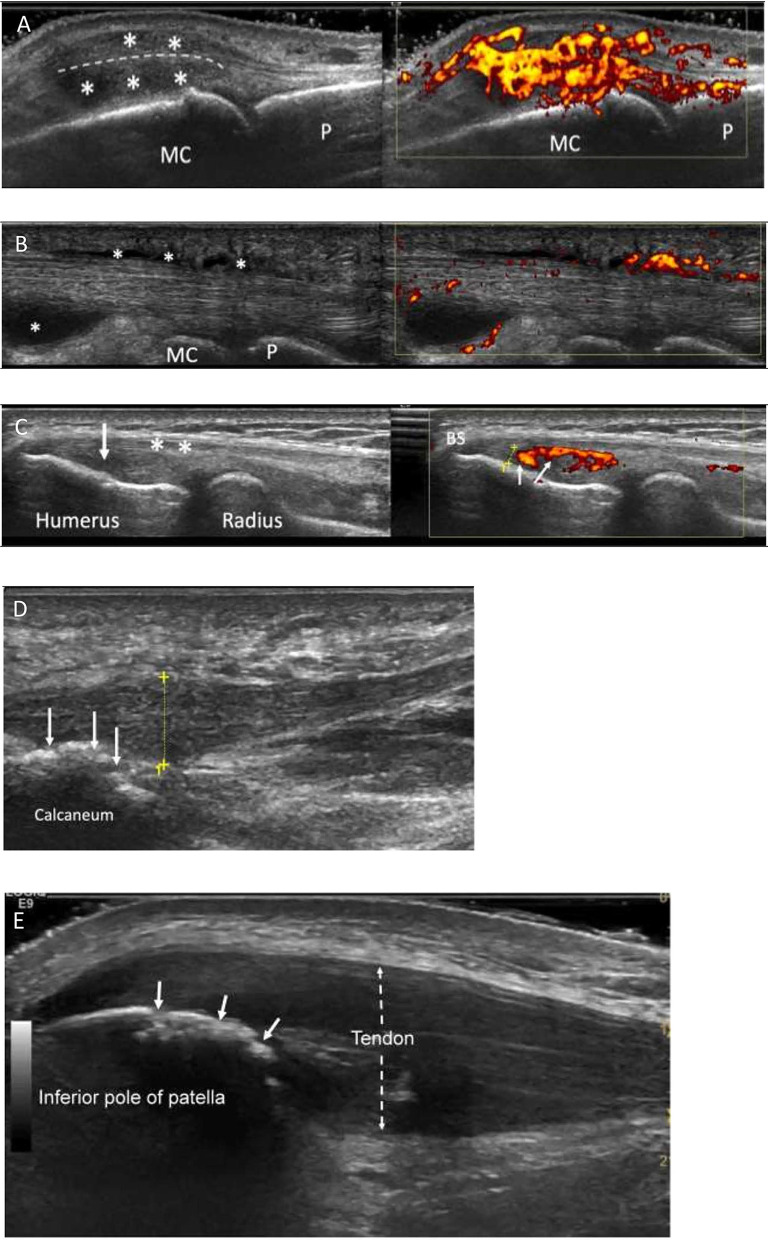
Characteristic ultrasound appearances in Psoriatic arthritis. (A) Longitudinal view through a metacarpophalangeal joint with synovitis. There is gray scale thickening (*between joint and extensor tendon-dotted line) and marked increased power Doppler signal (grade 3, right image) consistent with “active” synovitis and peri-tendonitis (*above tendon). MC, metacarpal; P, phalanx. (B) Longitudinal view through the flexor tendon on a finger. It demonstrates fluid and synovial thickening within the tendon sheath (*). There is also marked power Doppler signal within the tendon sheath (right image). MC, metacarpal; P, phalanx. (C) Enthesitis of common extensor origin (CEO): Longitudinal view through the common extensor origin. Hypoechogenicity (arrow/left), loss of fibrillary pattern (*), Bone spur (BS), increased Doppler signal (small arrows/right) within 2 mm (dotted line) of bone surface. (D) Longitudinal view through the plantar fascia of a patient with PsA. There is thickening of the fascia (dotted yellow line measuring 8.3 mm; normal < 5 mm). Power Doppler signal is rarely seen at the plantar fascia insertion. Bone irregularity is suggestive of erosive change (arrows). (E) Longitudinal section through the proximal patellar tendon. The tendon is markedly thickened (dotted arrow), hypoechogenic (compared to normal tendon more distally) and has lost its fibrillary pattern. The bone surface of the patella shows bone irregularity consistent with erosive change (white arrows). This was confirmed on transverse view.
